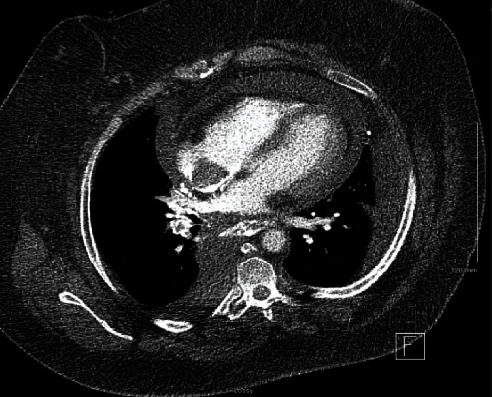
CT of the chest with right atrial thrombus and pericardial effusion.
